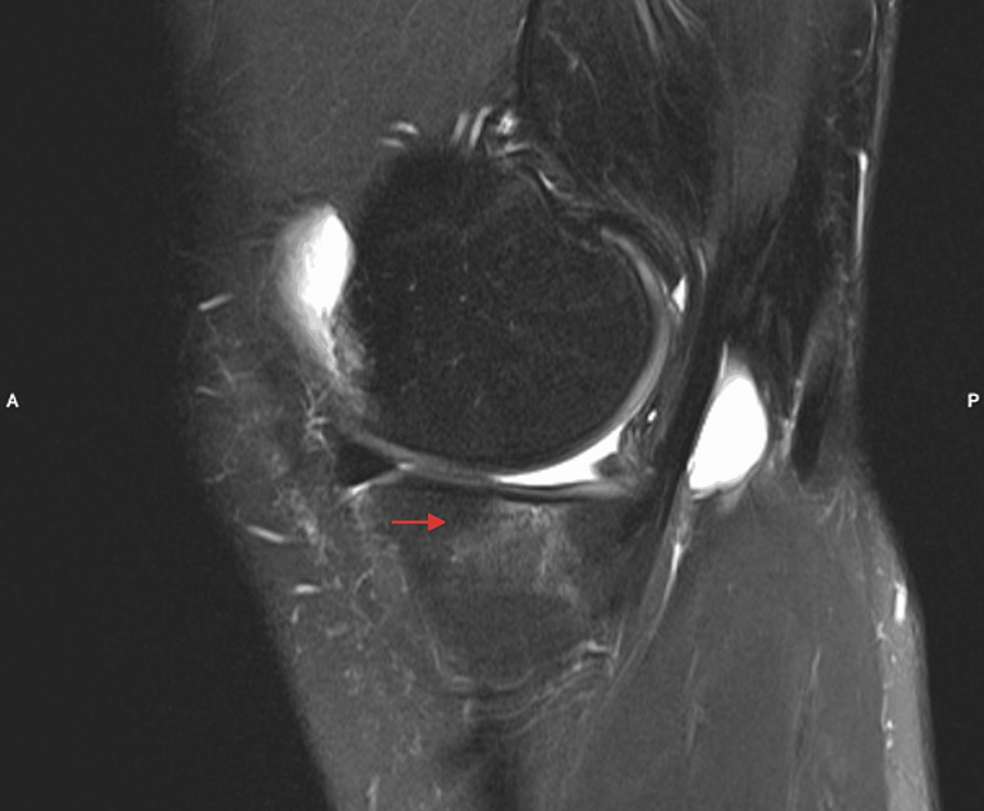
Pre-MAT MRI Status post partial medial meniscectomy. Bone marrow edema (red arrow) and increased signal intensity in the remaining meniscus. Sagittal view of the left knee, medial meniscus. MAT: Meniscus allograft transplantation; MRI: magnetic resonance imaging
